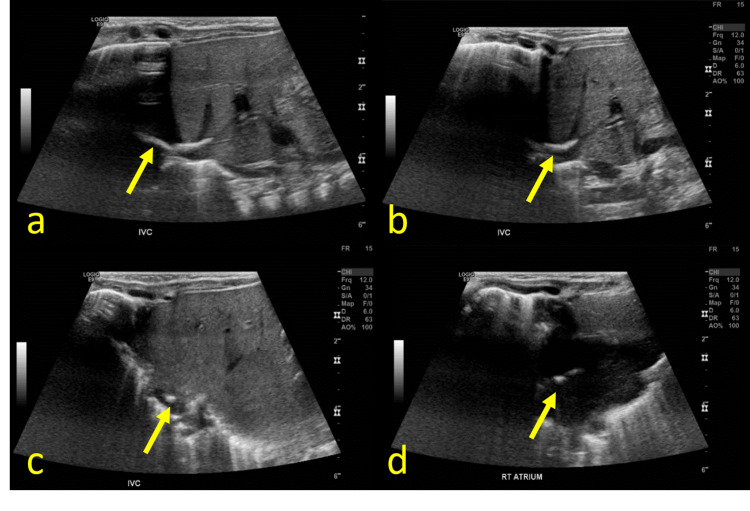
Grayscale transverse (a, b) and axial (c, d) ultrasound images of the right upper quadrant demonstrates a non-occlusive curvilinear hyperechoic structure extending from the confluence of the left hepatic vein and ductus venosus through the intrahepatic IVC to the right atrium (arrows) IVC: inferior vena cava
